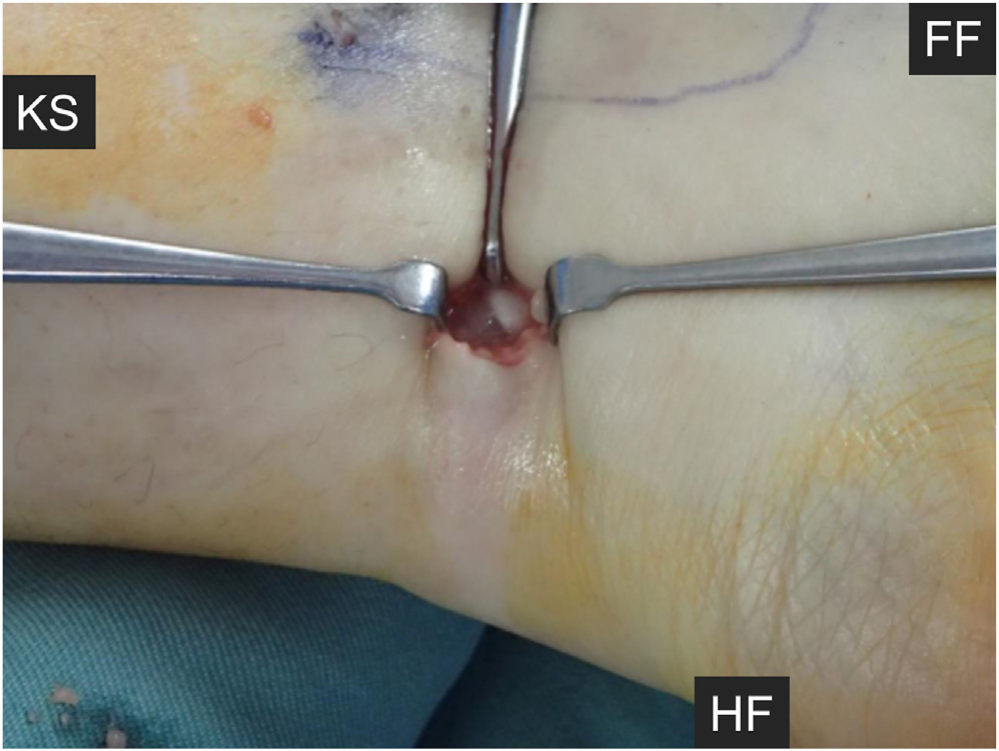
Exposure of flexor hallucis longus tendon of the left foot under direct vision. The tendon is hooked using a blunt instrument after blunt dissection under ultrasonography and then exteriorized through the skin incision for tenotomy. (FF, forefoot side; HF, hindfoot side; KS, knee side.)
